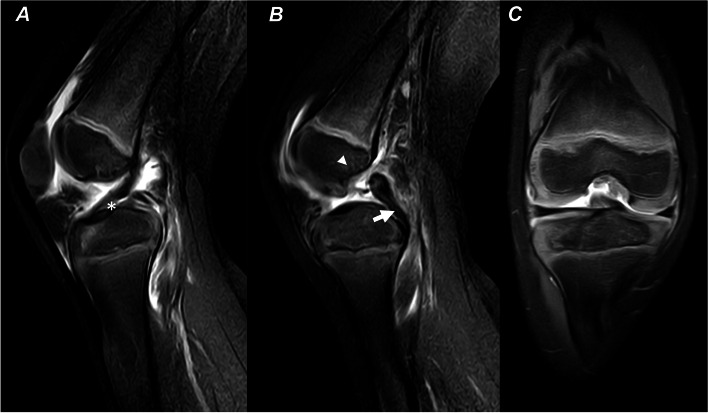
Pre-operation MRI showing an intact ACL (a), a complete proximal tear of the PCL (b), and intact menisci (c). ACL (asterisk); PCL (arrow); Medial condyle (arrowhead)
